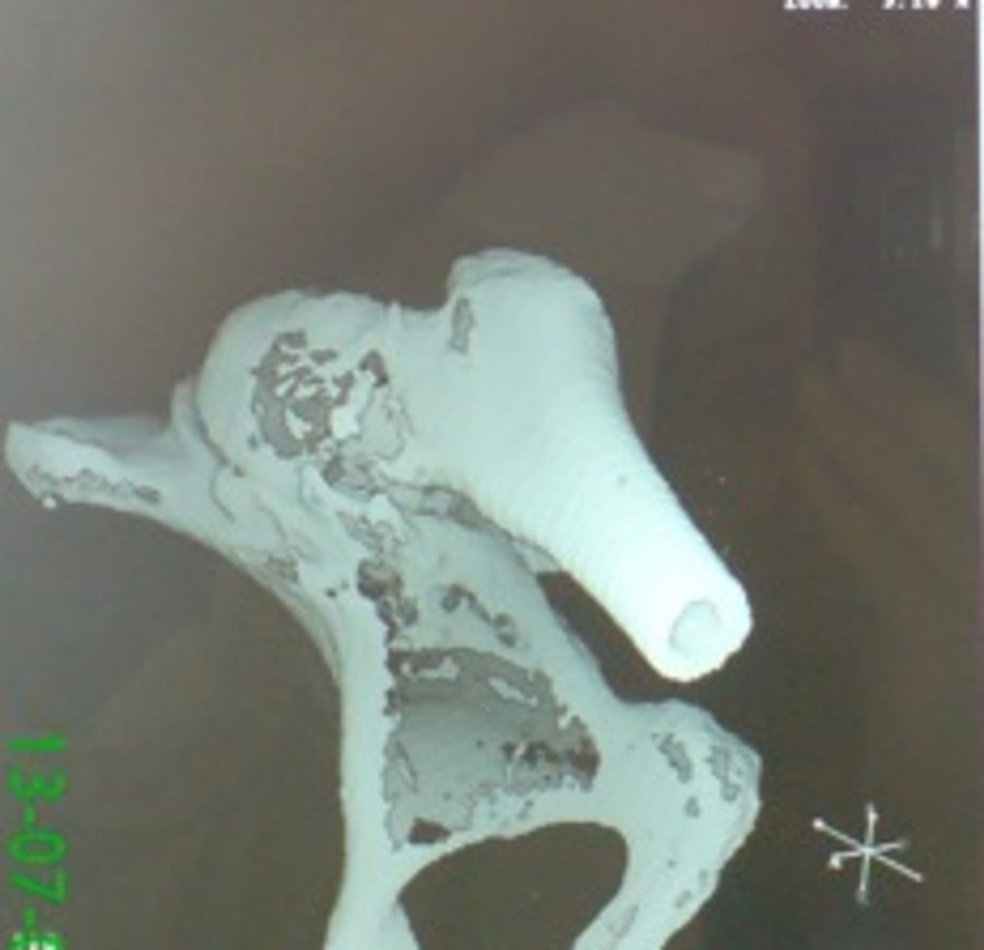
3D hemipelvis remodelling from CT scan of dysplastic hip.
